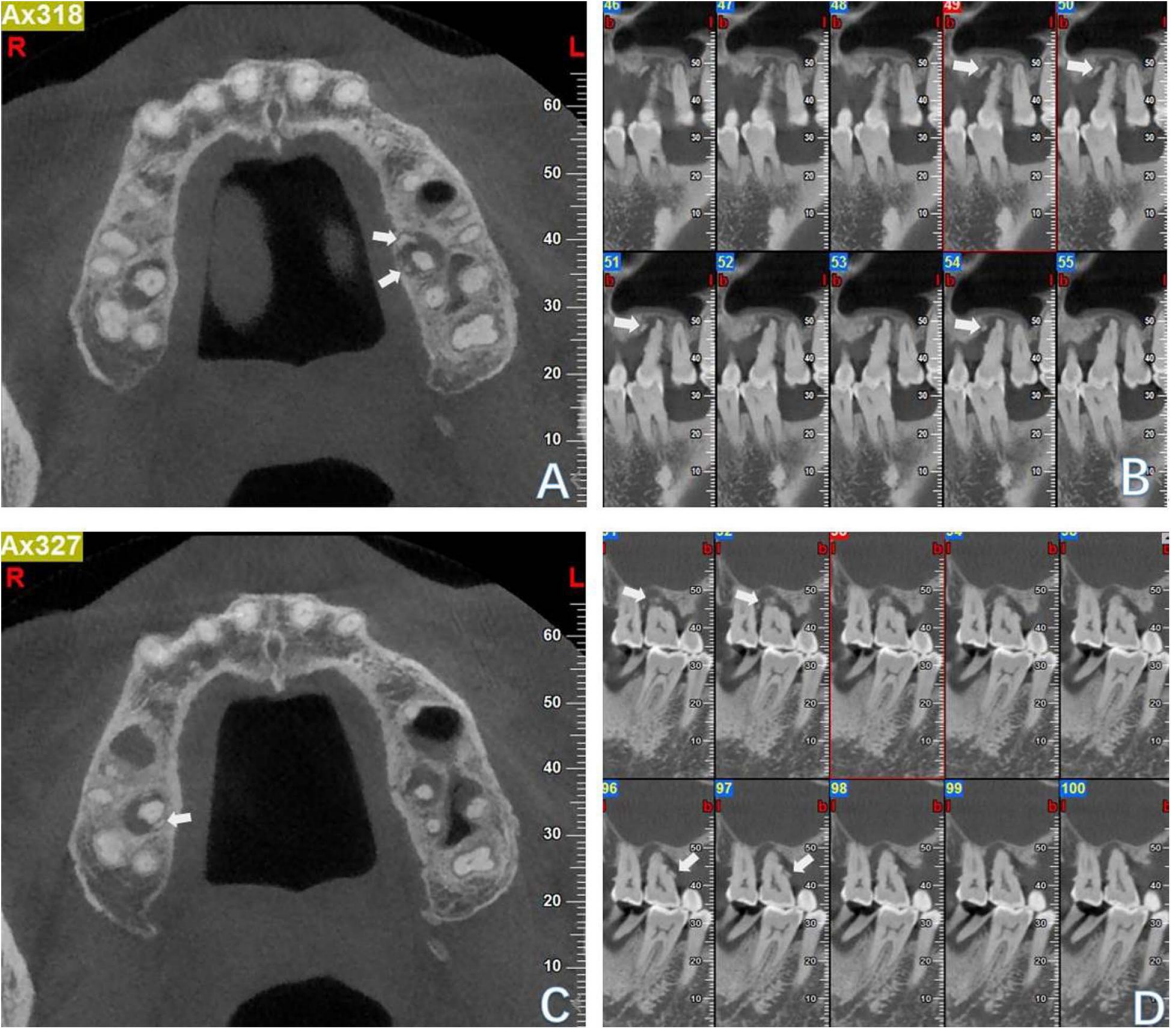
CBCT images of the left maxillary first molar (A,B) and the right maxillary first molar (C,D) in axial and sagittal views (arrows indicated the cemental tear).
